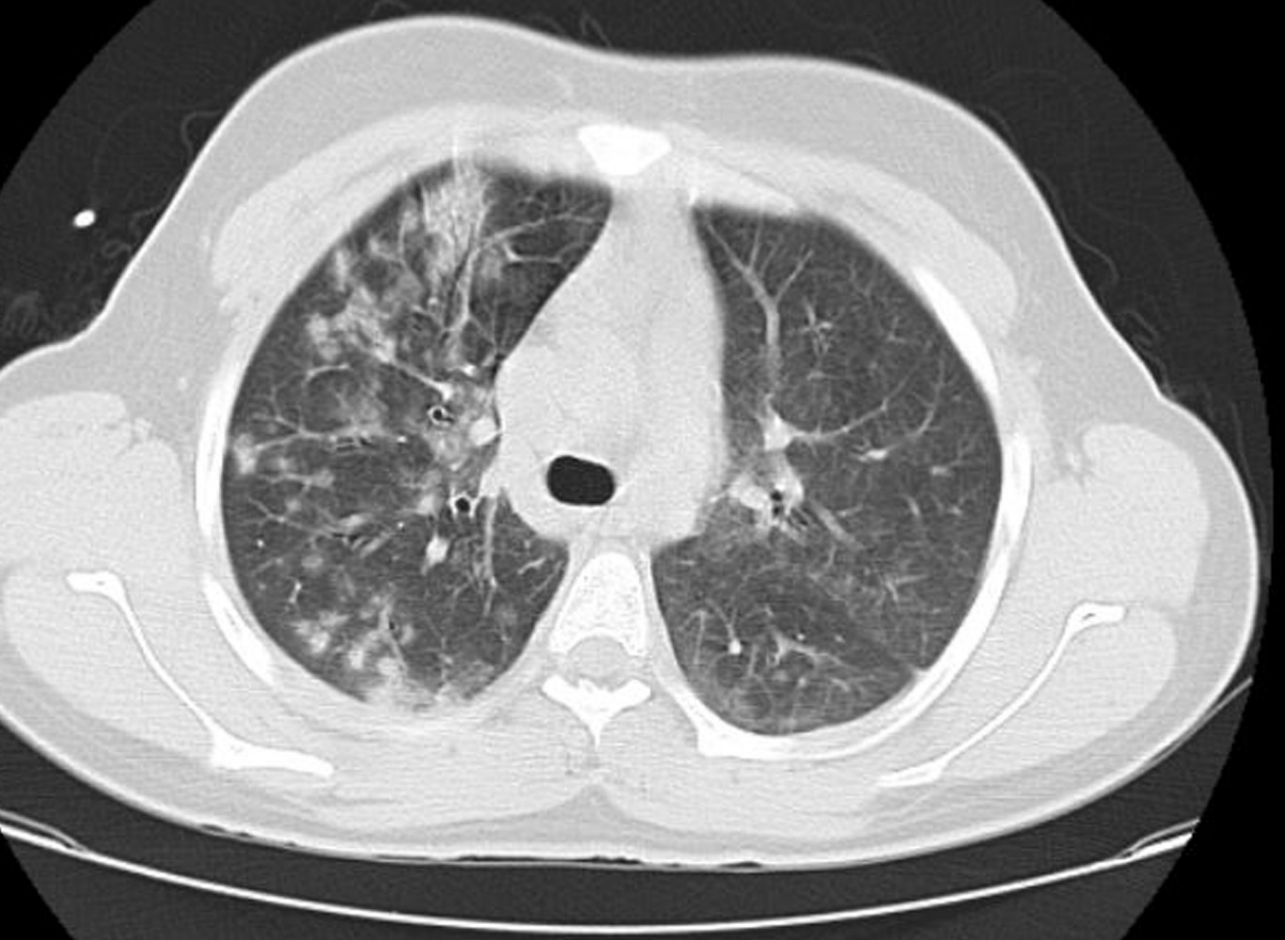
Chest CT of patient number 5.
